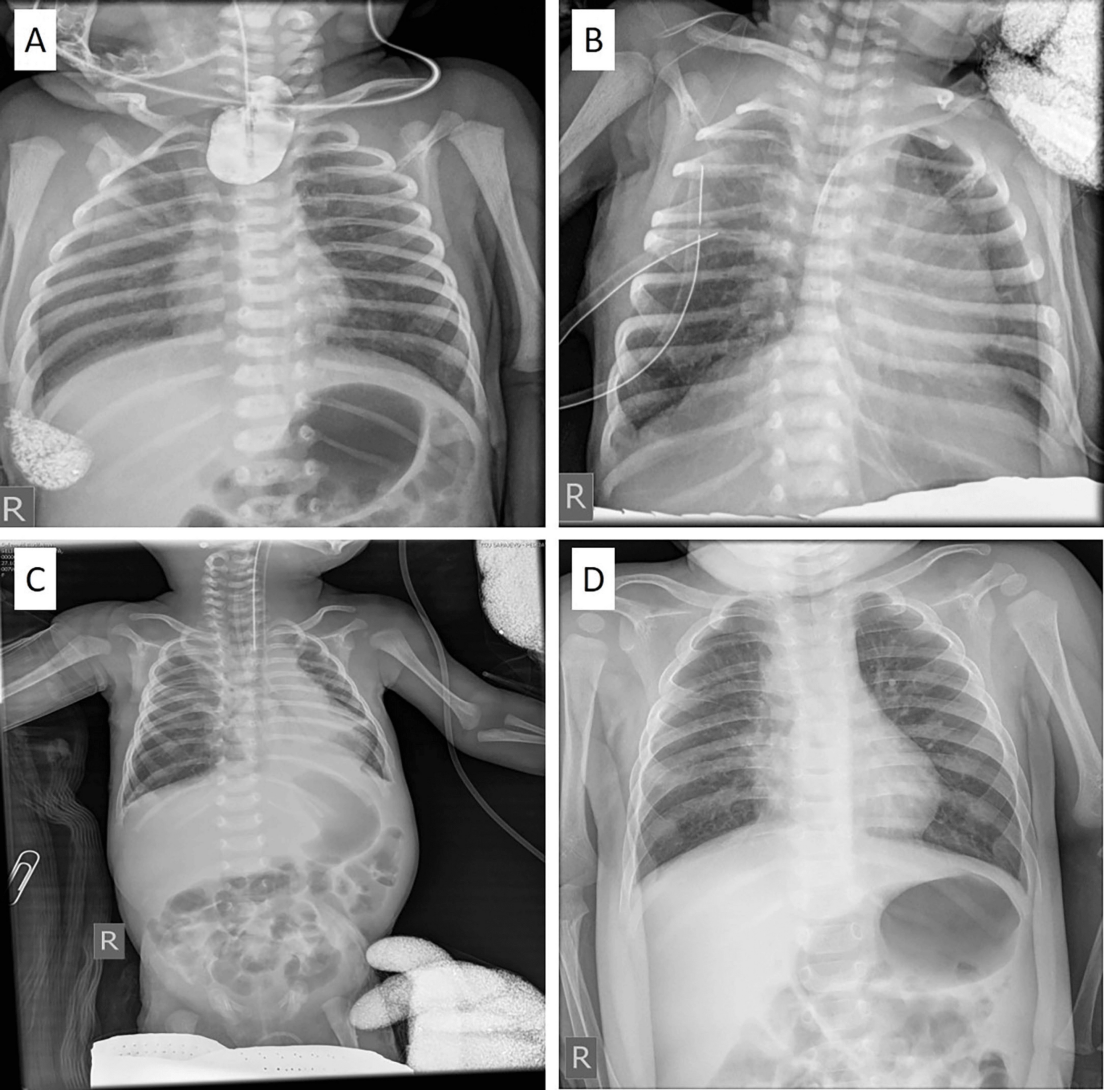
Chest X-rays (A) Preoperative chest X-ray of a neonate showing water-soluble contrast material (iodixanol) in the blind-ending upper esophageal pouch at the third thoracic vertebrae (Th3) with the air in the stomach and intestine; (B) Chest X-ray performed three days after primary esophageal repair showing two chest tubes on the right side for drainage of abundant pleural effusion; (C) Chest X-ray performed two days following open thoracic duct ligation showing the resolution of pleural effusion; (D) Chest X-ray performed seven months after the operation showing bilaterally normal chest finding
